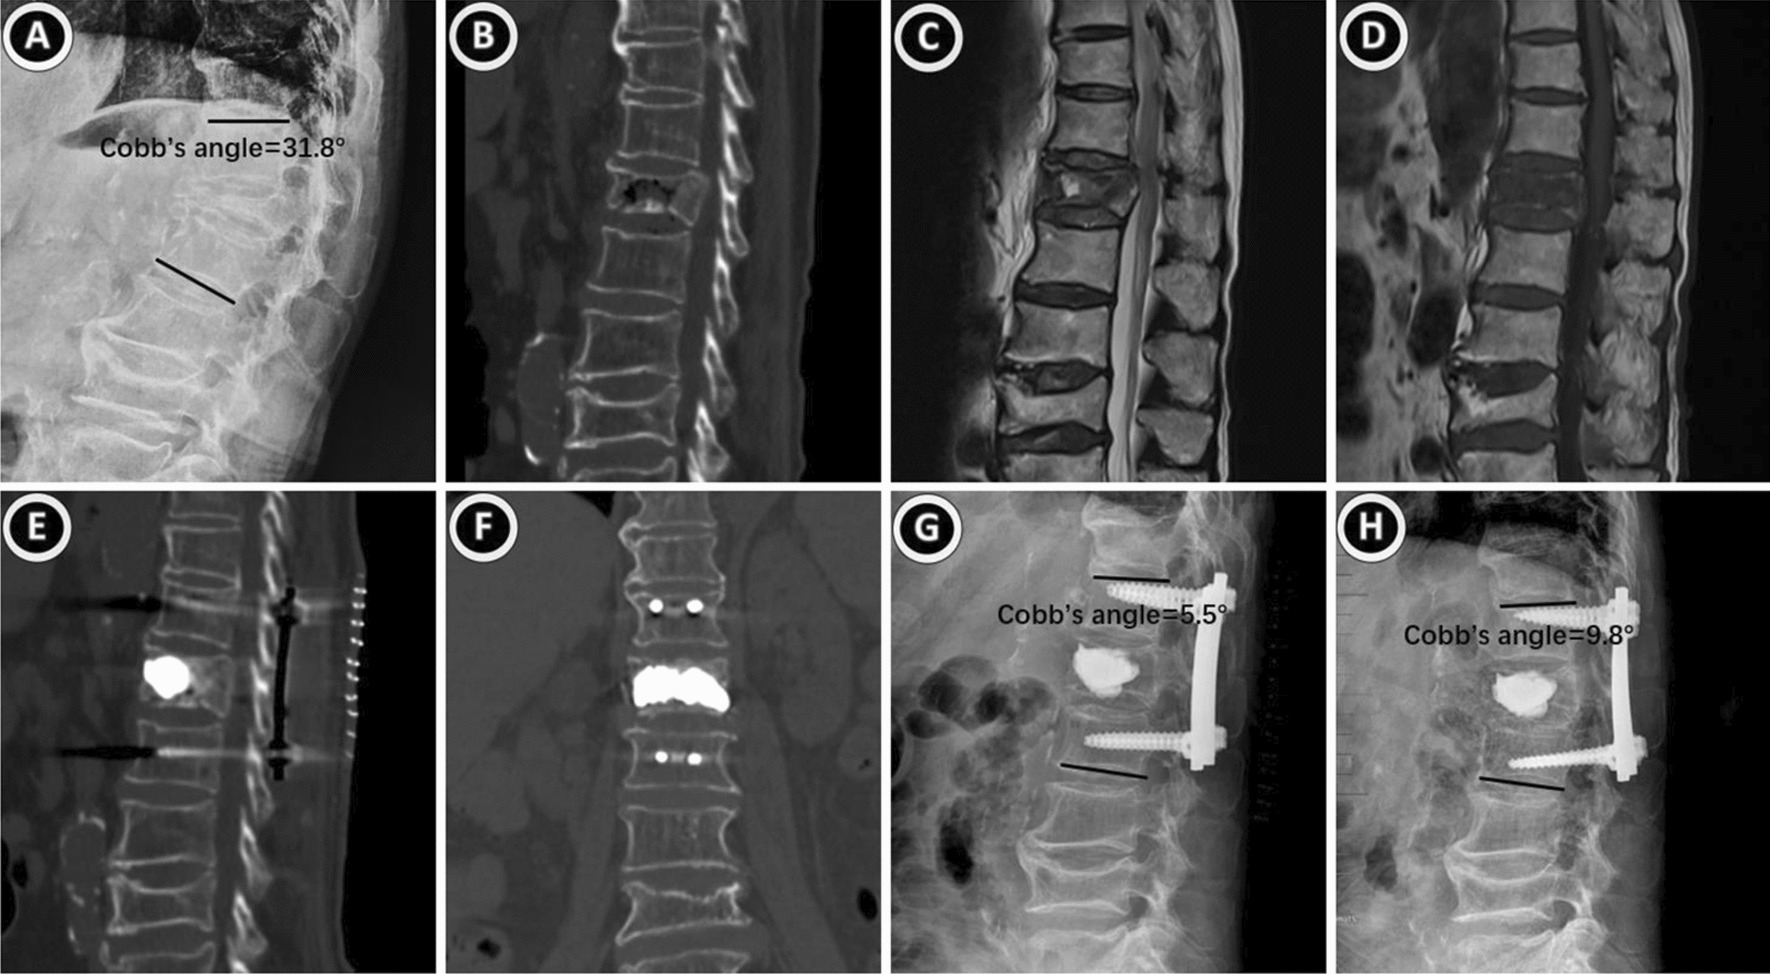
An 89-year-old woman who had L1 sOVCF with nonunion was treated with PPSF + KP. A–D The preoperative X-ray, CT and MRI showed a severe osteoporotic vertebral compression fracture with nonunion. E, F Her postoperative CT scan revealed that bone cement was evenly distributed throughout the vertebral body. G, H The postoperative and final follow-up X-ray displayed the vertebral height and the Cobb’s angle was well recovered
